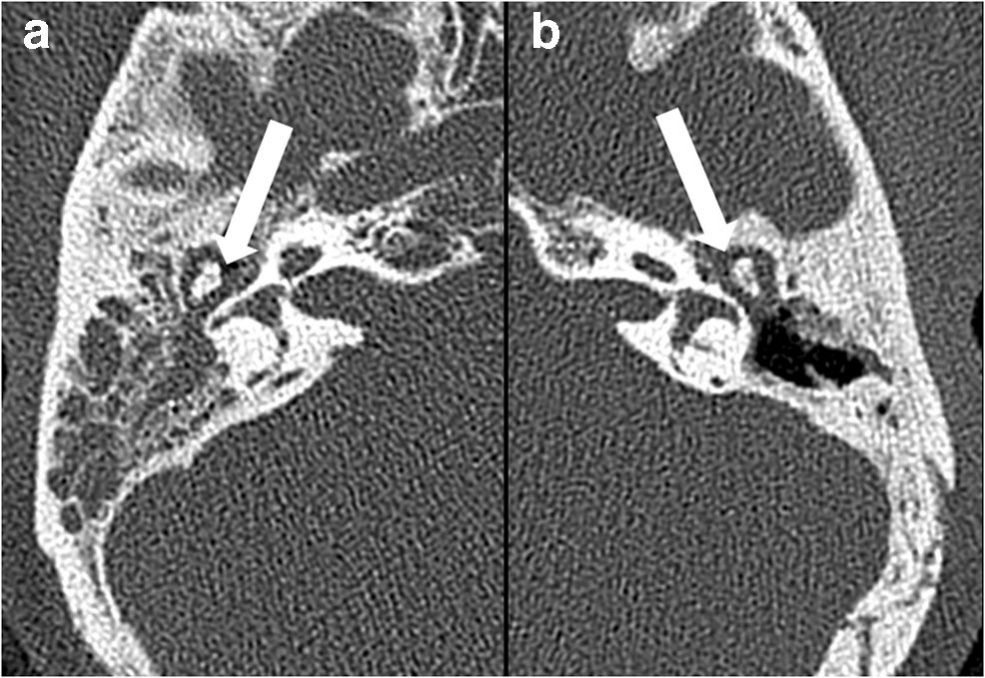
Left: unenhanced high-resolution computed tomography (HRCT) image of a 54-year-old female patient with middle ear inflammation (MEI) in the right tympanic cavity (a). Right: unenhanced HRCT image of a 19-year-old male patient with a cholesteatoma in the left tympanic cavity (b). Both show soft tissue in the middle ear without bone destruction. Radiomics characteristics derived from unenhanced HRCT-differentiated MEI from cholesteatoma with an overall median area under the receiver operating characteristic curve (AUC) of 0.78 (separate accuracies: center A, 66%; center B, 84%) in our patient collective. Post-processing in terms of data resampling and harmonization yielded overall median AUCs of 0.88, and 0.89, respectively
